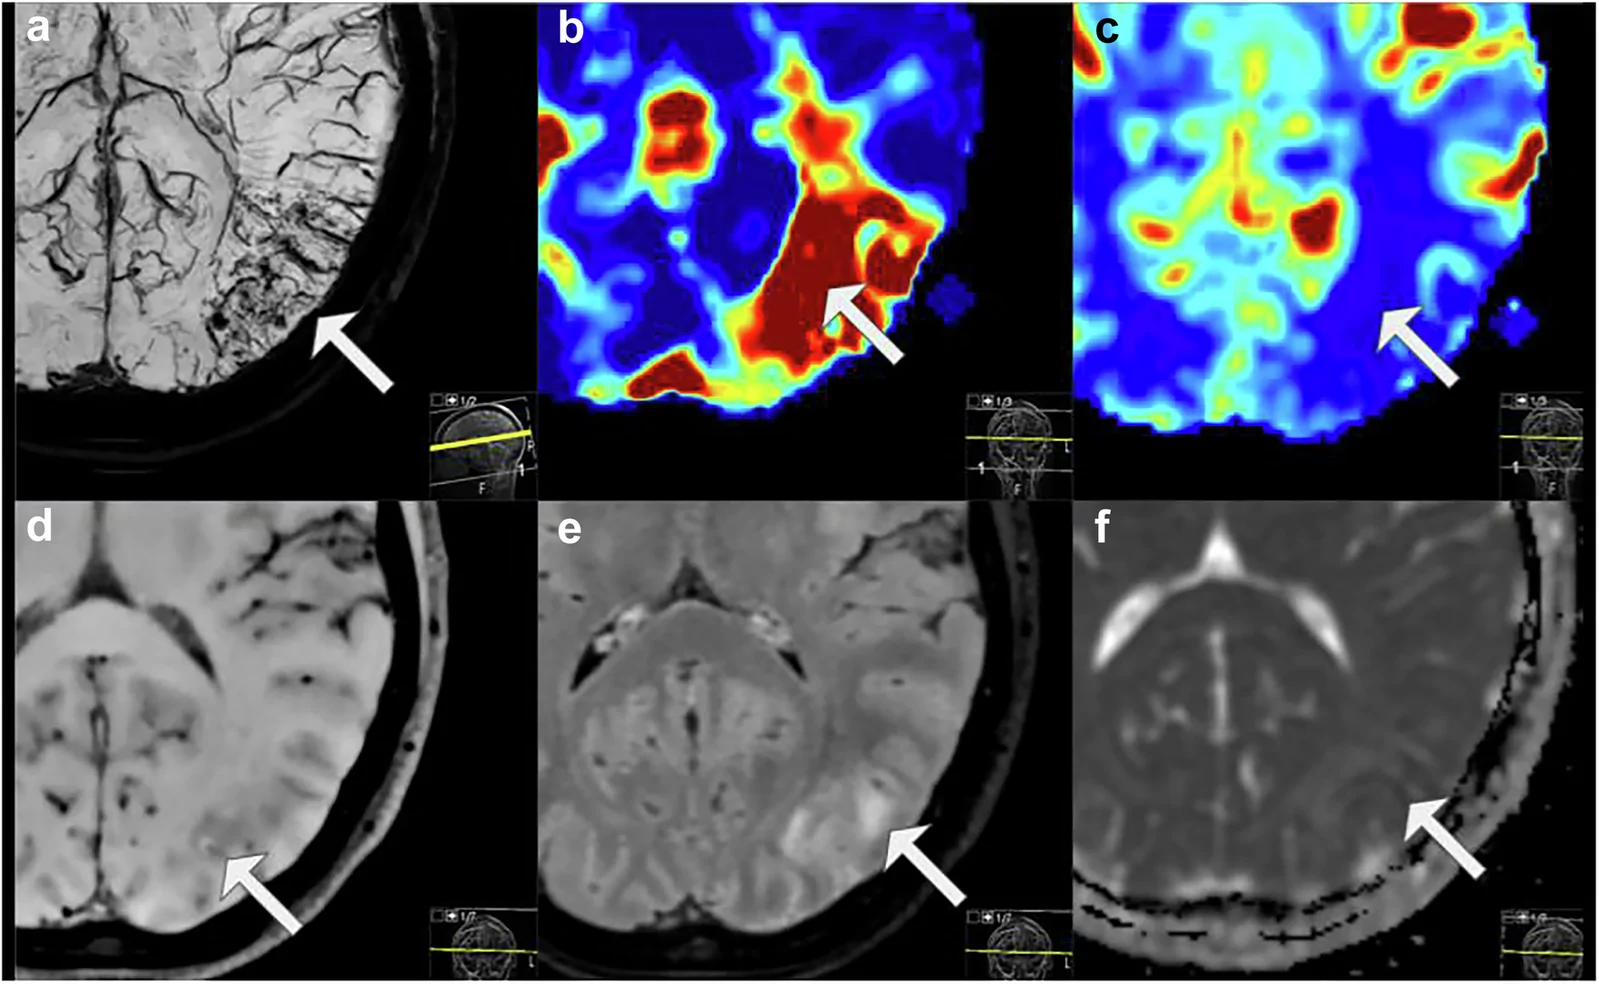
Extensive SSS and left transverse sinus thrombosis with multiple complications. a SWI shows corkscrew-dilated medullary veins (arrows) and microhemorrhages. b, c Perfusion imaging demonstrates prolonged MTT and reduced CBF in the left hemisphere. d Post-contrast T1 CUBE FS reveals subtle cortical hemorrhage (arrow). e T2-FLAIR shows vasogenic edema. f ADC map demonstrates focal cortical restricted diffusion (arrows), indicating mixed vasogenic and cytotoxic edema
